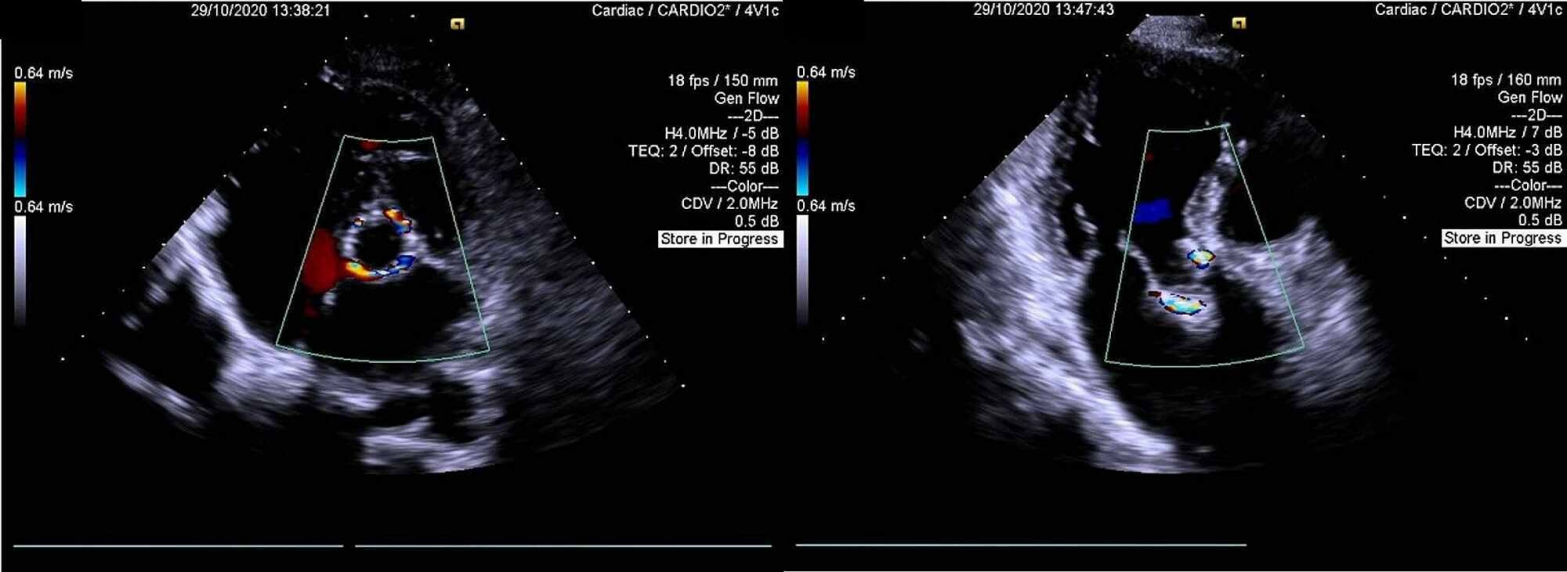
Pre-discharge transthoracic echocardiogram A parasternal short-axis view at the aortic valve level is shown in the left panel. An apical long-axis view is shown in the right panel.
